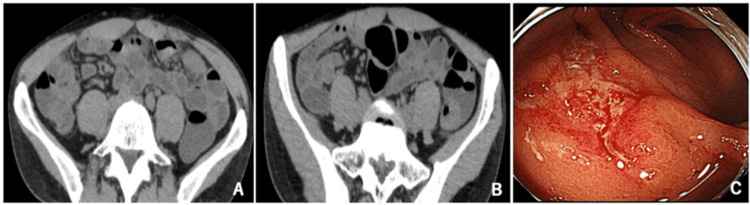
CT and CS findings after the second hospitalization A, B: CT findings showing mild wall thickening in the ileum, wall thickening in the entire colon, and fluid retention in the colon. C: CS findings revealed that the rectal ulcers tended to regress, and there was no obvious abnormality other than in the rectum.
